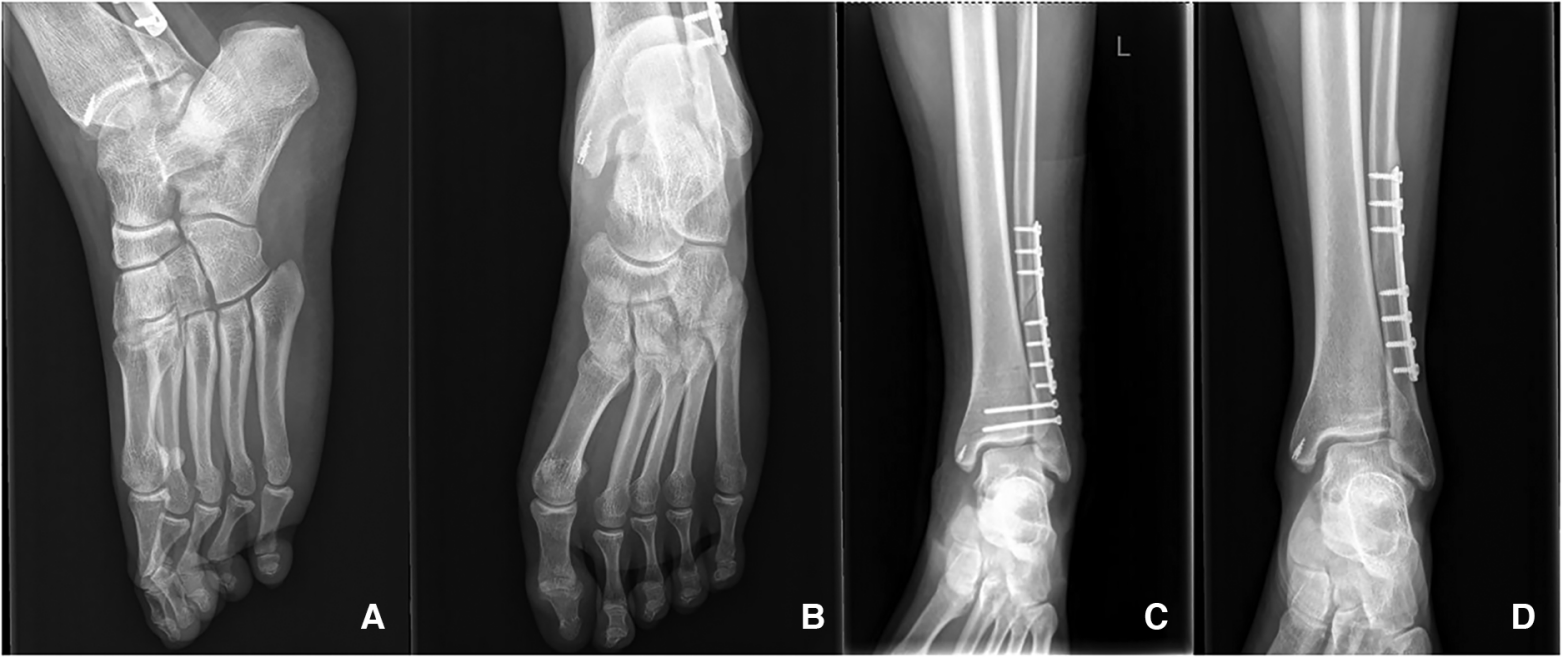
X-ray of the left foot and ankle 32 months after the primary operation. No dislocation of the IP and MP joints (A,B) and synostosis formation at the fracture site or interosseous membrane (D) was observed. Syndesmotic screws (C) were removed at postoperative 10 weeks after primary operation.
